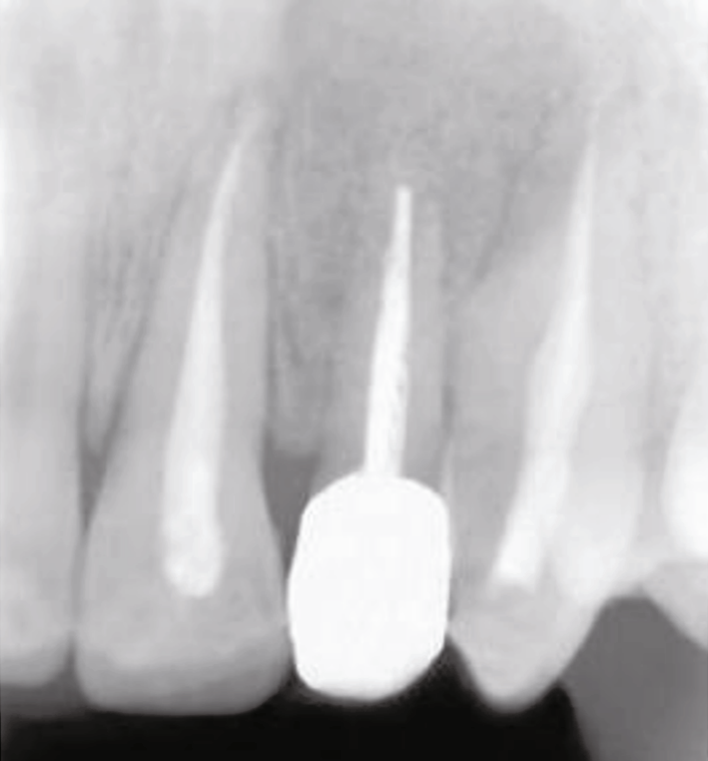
X-ray at 4-year follow-up.
